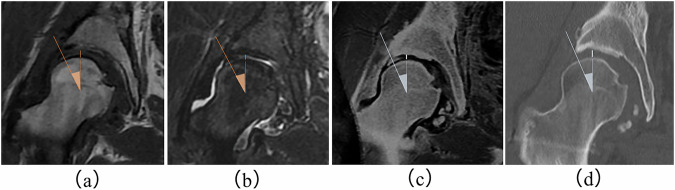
Representative clinical case. A 23-year-old female patient underwent MRI and CT examinations several months after right hip PAO. On coronal images: (a) T1WI shows a JSW of 4.9 mm; (b) STIR shows 3.6 mm; (c) MERGE shows 4.0 mm; and (d) CT shows 4.2 mm. The CE angles were: 36° on T1WI, 33° on STIR, 30° on MERGE, and 30° on CT. CT, Computed tomography; MERGE, Multi-echo recombined gradient echo; STIR, Short tau inversion-recovery; T1-WI, T1-weighted imaging
